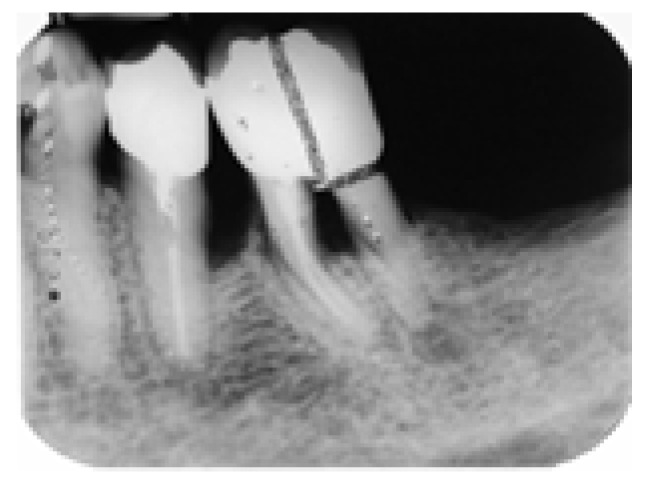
Initial periapical X-ray of tooth #36.
